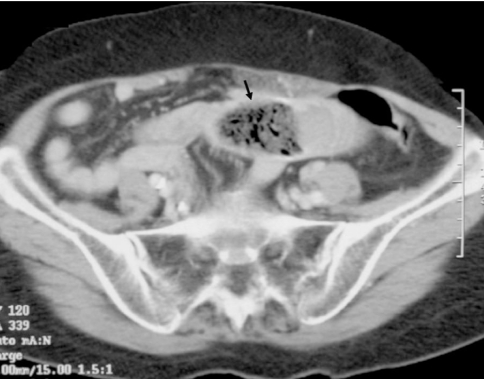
CT scan of the abdomen showing the intraluminal hypodense filling defect with mottled appearance (arrow).
